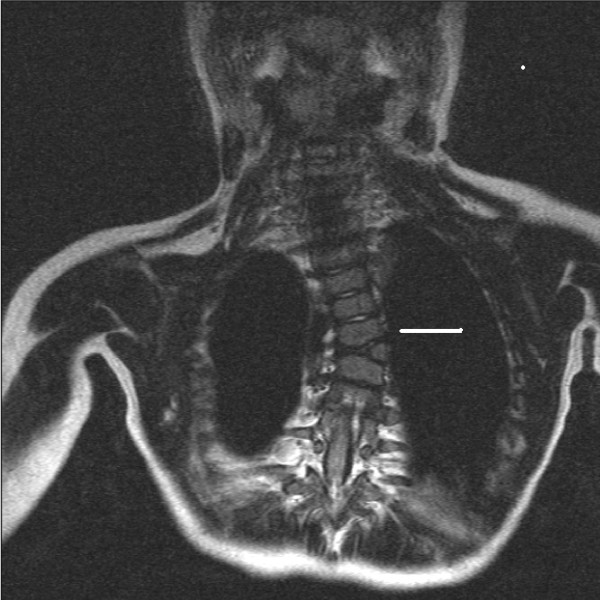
MRI T2-weighted Sequence, Coronal Plane Image. Congenital Scoliosis from Hemivertebra Patient # 21. MRI demonstrates convex zone deficiency in the vertebral body growth plate.
